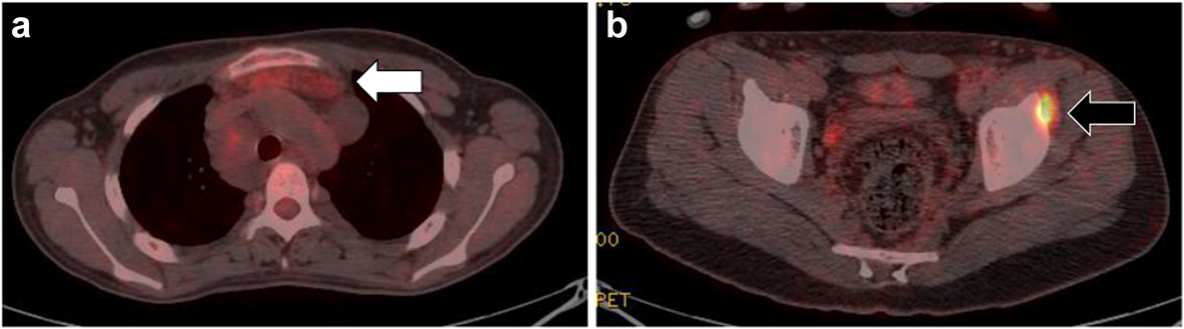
Axial FDG PET/CT image (a) showed that the FDG uptake (*white arrow*) in the mediastinal mass was mild or low-level (a) and high uptake (*black arrow*) in the extra-articular soft tissue mass of the left hip (SUVmax = 12.8 g/ml) (b)
